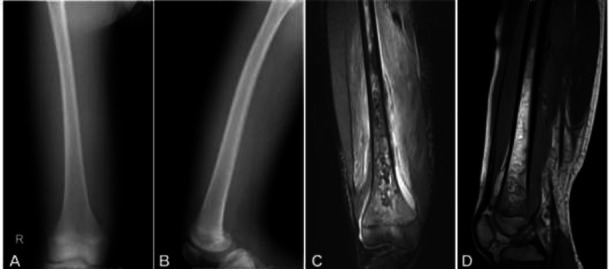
X-ray angiograms and magnetic resonance imaging (MRI) of the right femur. (A & B). Anteroposterior and lateral X-ray angiograms showed intact bone structure of the femur, without abnormal bone density, but with thickening of soft tissue and increased density of subcutaneous fat layer, which had a blurred boundary with muscles. (C & D). Coronal fat suppression (FS) MRI T2WI (C) and sagittal T1WI (D) of the right femur demonstrated patchy and tortuous mixed abnormal signals within the femoral bone marrow cavity and uneven and thinning cortical destruction in the lower femur. Diffuse high uneven signal shadows were shown within and between the muscles and in the subcutaneous fat layer in the right thigh. Abnormal grid-like signals were observed in the subcutaneous fat layer, and pus was revealed to surround the femur as high fluid signals.
